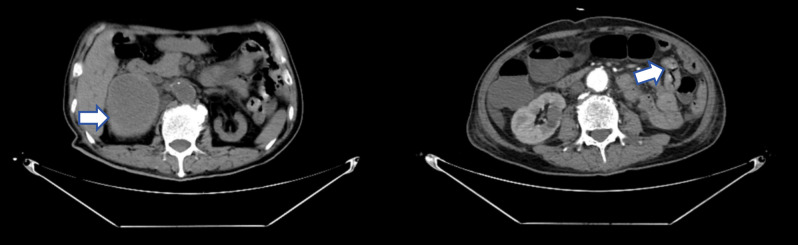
Abdominal CT image before surgery. A mass with a maximum cross-sectional size of approximately 4.0cm×3.0cm can be seen in the proximal jejunum, with mild enhancement. A mass shadow can be seen in the right adrenal area, with the maximum cross-sectional size approximately 12.3cm×8.4cm, and mild heterogeneous enhancement.
